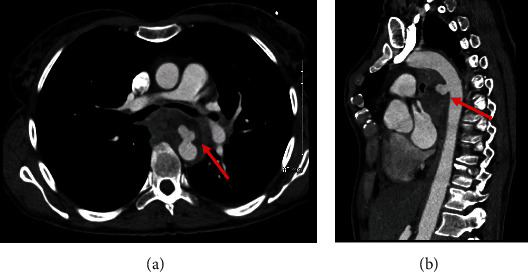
Axial (a) and sagittal (b) CT angiogram images demonstrating extravasation of contrast anteriorly into the surrounding esophageal tumour.
